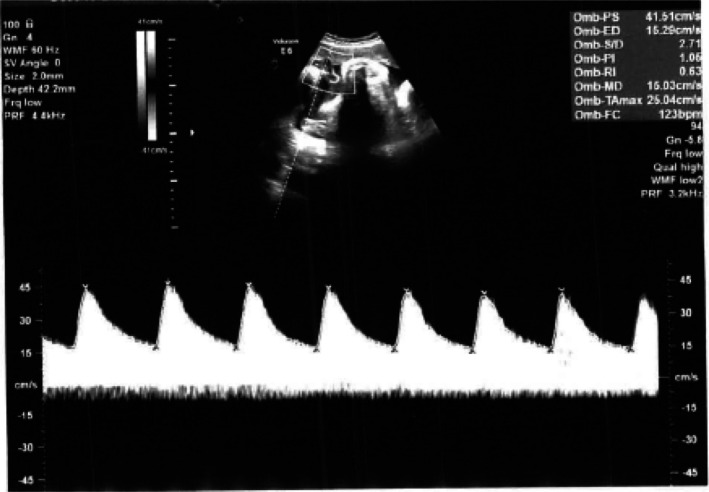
Ultrasound performed with a transabdominal probe shows a fetal umbilical artery pulsatility index of 1.06, demonstrating that good fetal tone and relative well‐being in the womb are maintained.
